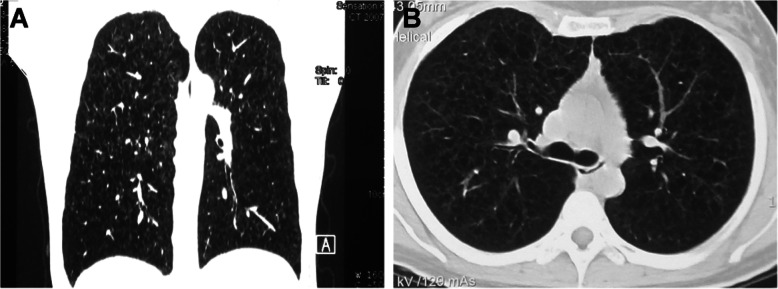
Thoracic CT findings of patients with LAM. a Coronal reformatted image of high-resolution chest CT images in a patient with LAM shows diffuse cysts; b Axial high-resolution chest CT images through the upper lungs
